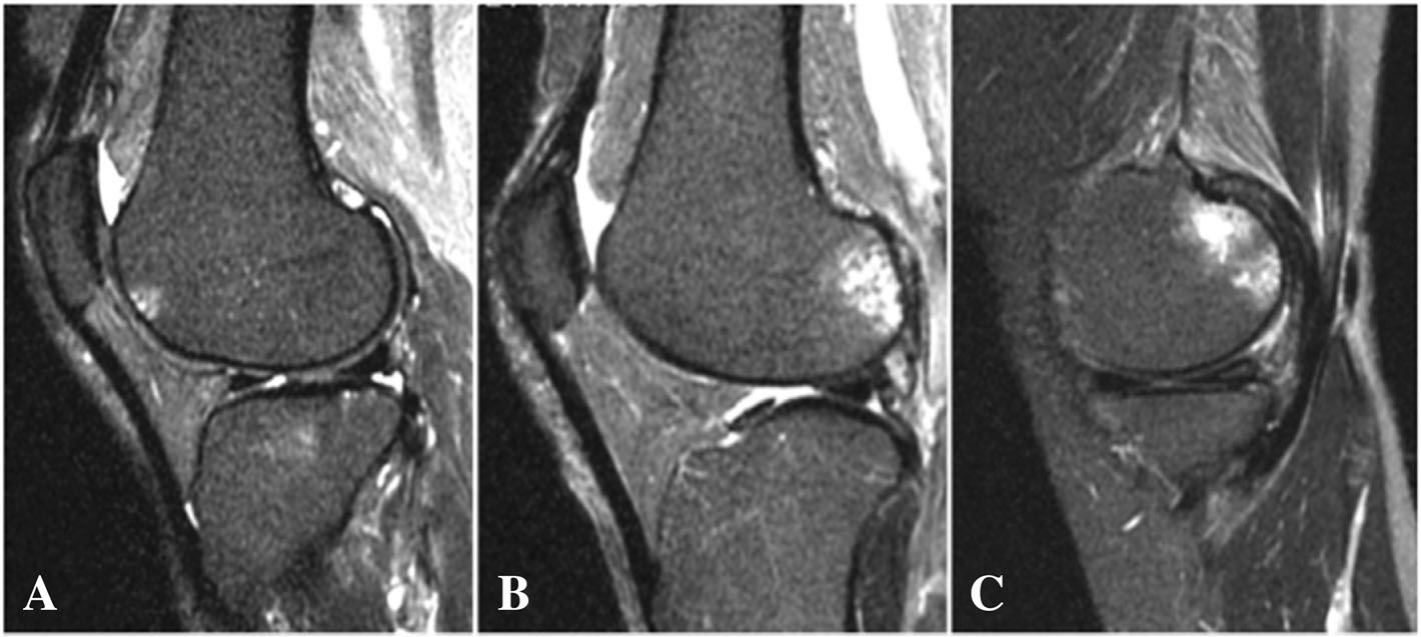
Evaluation of bone marrow lesions (BMLs). BMLs were measured using the coronal and sagittal T2-weighted images and marked by the increased signal intensity area in the subchondral bone. BMLs were graded according to the percentage of the area according to the Whole-Organ MRI Scoring method. a Grade 1 BML in the anterior lateral femur. b Grade 2 BML in the posterior lateral femur. c Grade 3 BML in the posterior medial femur
